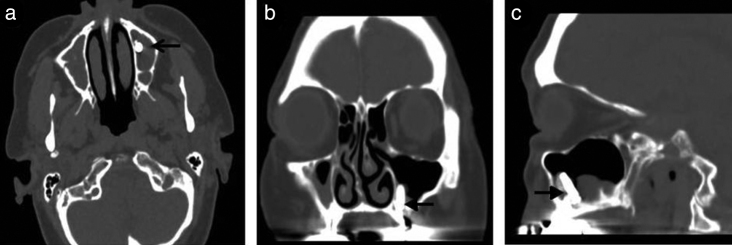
Computed tomography (CT) scan showed dental implant (arrow) inside left maxillary sinus in axial (a), coronal (b) and sagittal (d) view.
